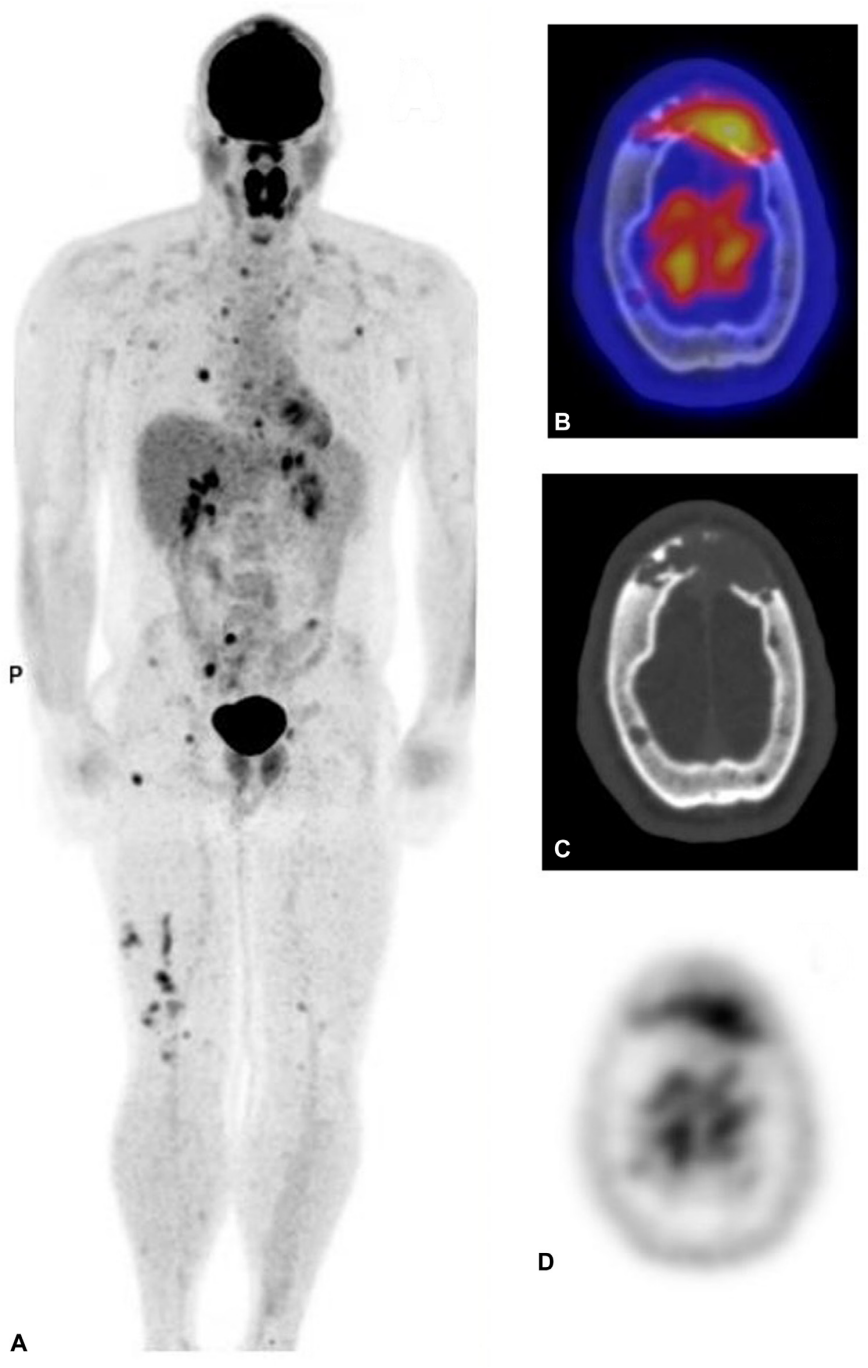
FDG PET scan. Maximum intensity projections showing distribution of hypermetabolic cutaneous, subcutaneous, intramuscular, and bony lesions (A). Large lytic lesion of the frontal bone with erosion of the inner and outer table of the calvarium seen on fused (B), CT (C), and PET (D) views. *FDG*, Fluorodeoxyglucose; *PET*, positron emission tomography; *CT*, computed tomography.
